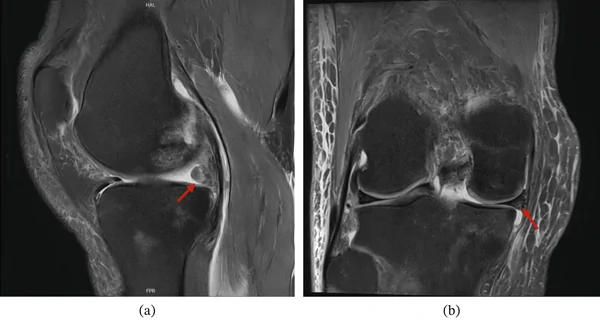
Sagittal and coronal MRI views of the right knee. (a) sagittal view showing the “ghost sign,” discontinuity of the medial meniscus into its posterior attachment. (b) coronal view, highlighting meniscal protrusion (marked by the arrows), suggesting an avulsion of the posterior root.
